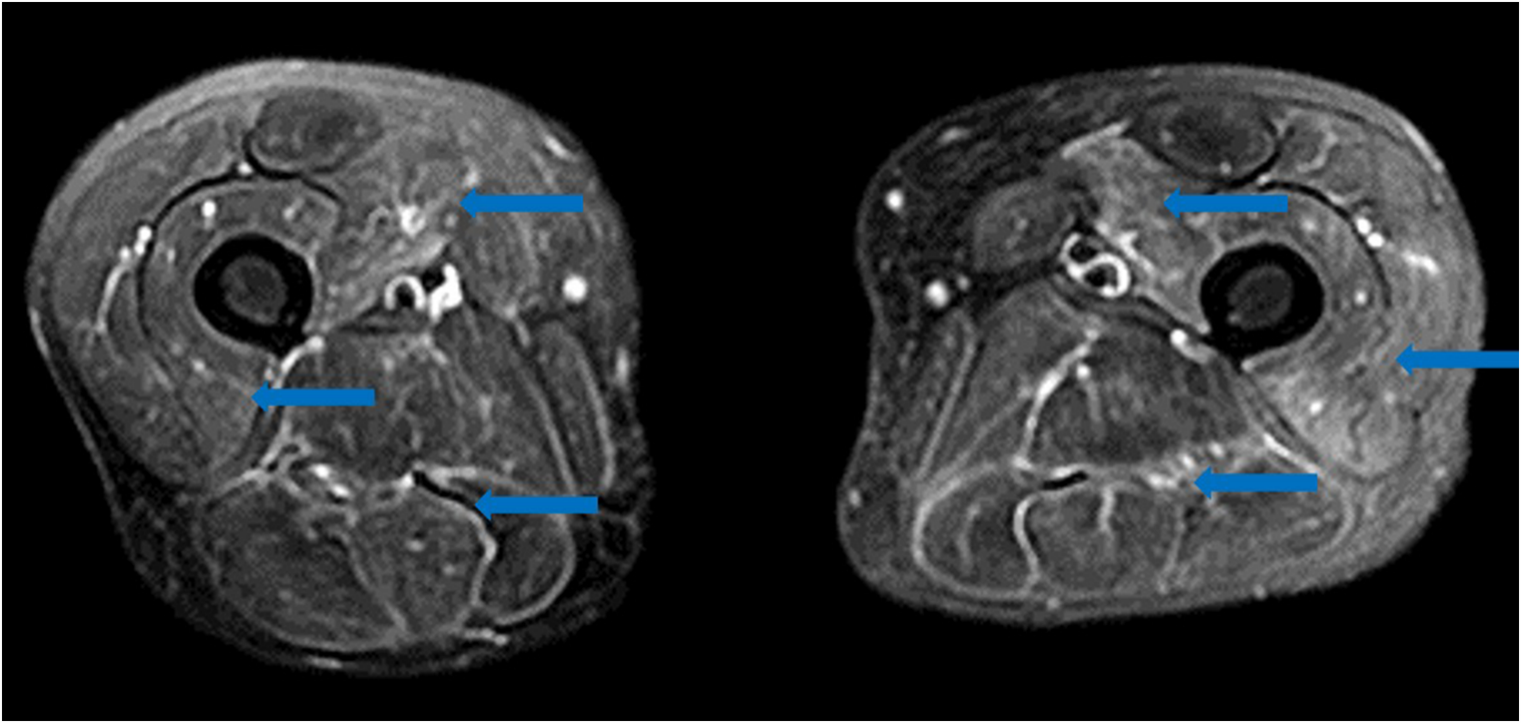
Axial fluid sensitive MRI of the thighs demonstrates diffuse multicompartment intramuscular high T2 signal, indicative of edema and myositis.
